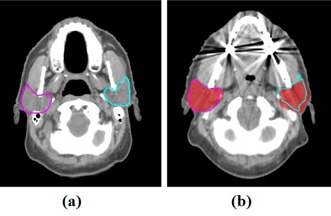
Parotid contours on the reference planning CT (a) was deformed to another patient using the dual‐force demons DIR algorithm. The deformed contours were compared with the manual contours (red colorwash) on this patient's planning CT (b).
